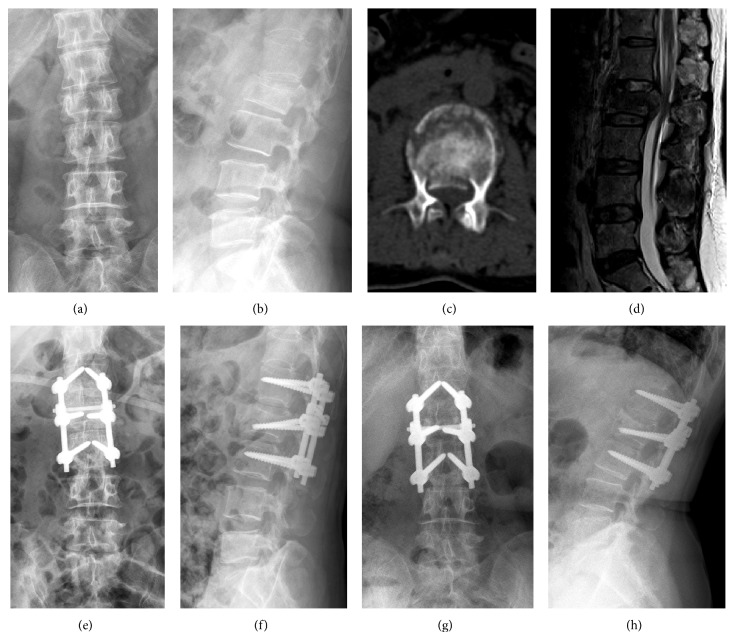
(a) and (b) Preoperative X-ray; (c) preoperative CT cross section; (d) preoperative MRI sagittal; (e) and (f) postoperative radiographs; (g) and (h) radiographs after 24 months.
